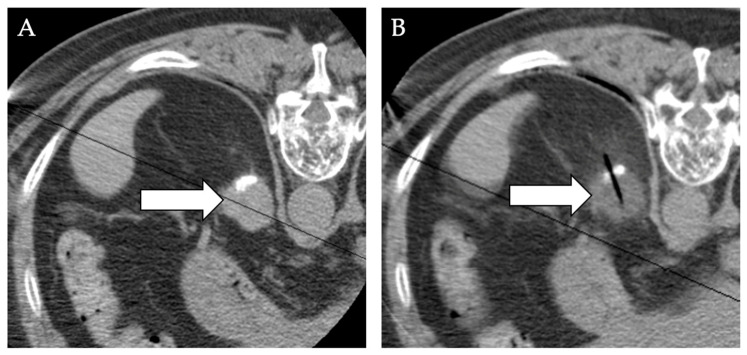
Cryoablation of adrenal tumor. (A) Axial CT image in an HCC patient with a single metastatic focus to the left adrenal (white arrow). (B) During cryoablation the entire mass (white arrow) demonstrates lowered HU as its density decreases during freezing. The “ice-ball” is difficult to visualize in fatty regions because its CT density is very close to that of fat. The peri-adrenal fat becomes “fuzzy” indicating extension of the “ice-ball” around the adrenal mass.
